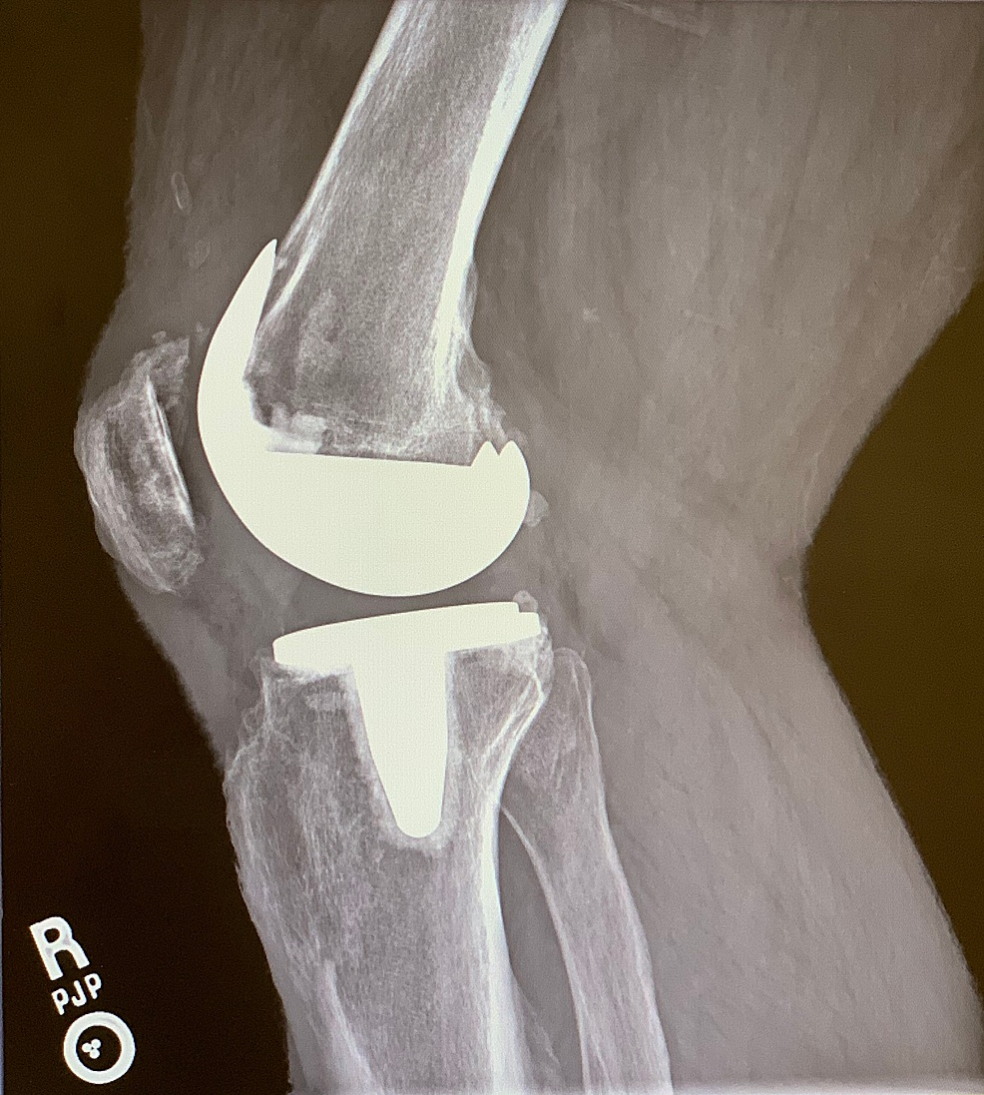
Preoperative lateral radiograph of the right knee demonstrating early anterior subsidence of the tibial baseplate.
